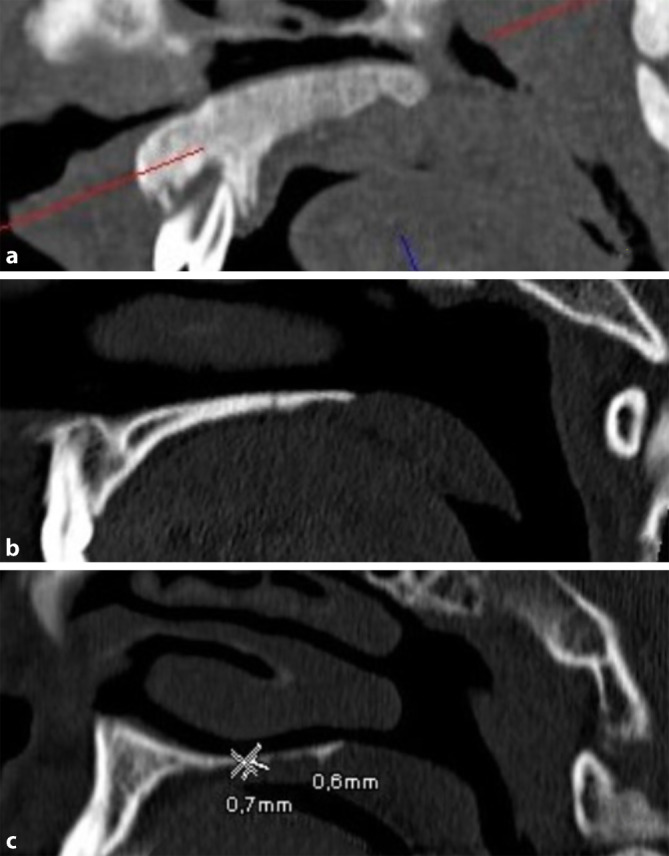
Example computed tomography images of hard palate thickness in patients with a osteopetrosis, b without bone disease, and c osteoporosis
